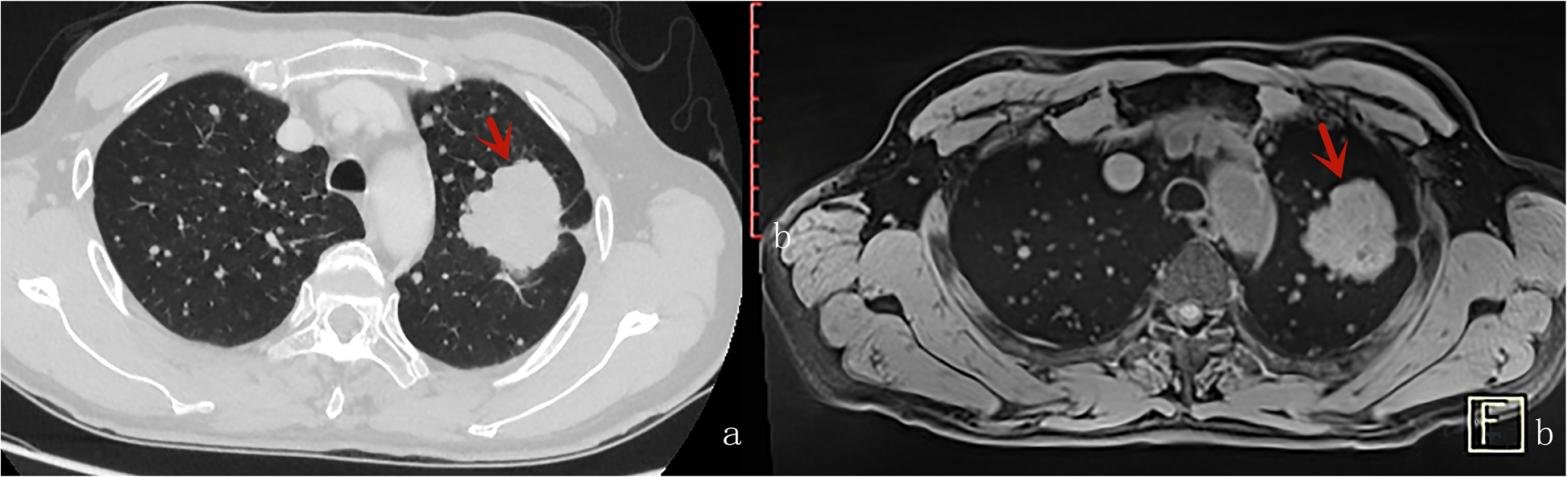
A male with adenocarcinoma, lobulation (the red arrow) and pleural indentation (the red arrowhead) were visible in axial CT(A) and MR (B)
